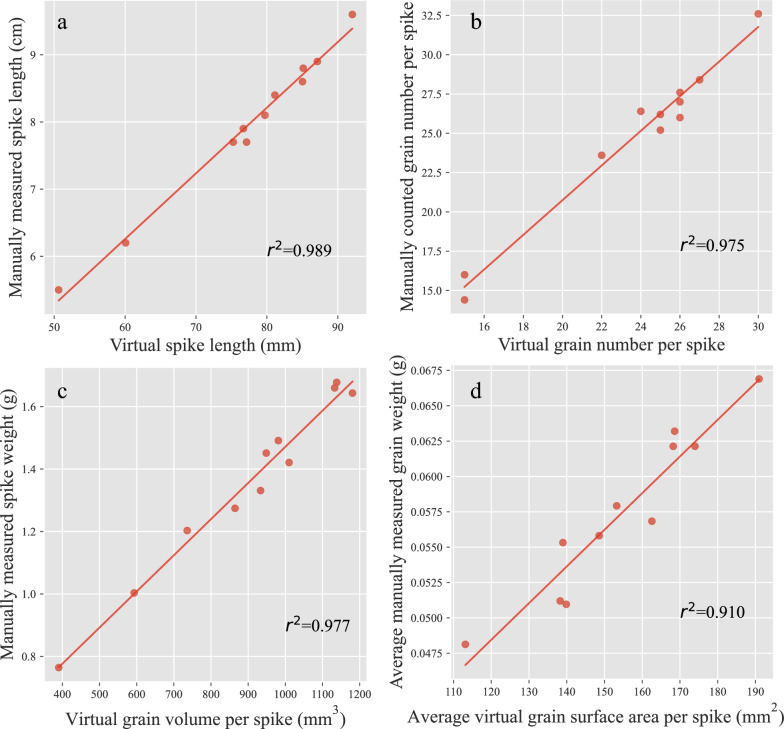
Validation of CT-based extraction of barley phenotypic traits. a Spike length. b Grain number per spike. c Relationship between spike weight and virtual spike volume. d Relationship between the spike weight and virtual spike surface area
